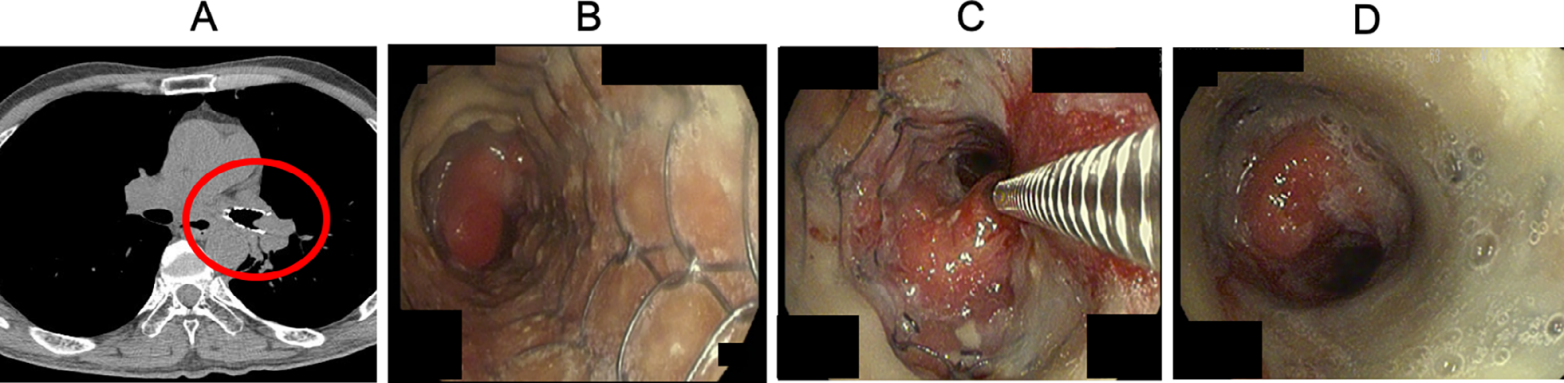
Chest computed tomography showing the narrowing airway at distal portion of the left main bronchus 2 years and 3 months after stent insertion (A). Bronchoscopic examination showing polypoid mass lesion at the distal end of the inserted stent (B). The procedure of bronchial ethanol injection (BEI) therapy with aspiration needle using absolute ethanol (C). Size reduction of polypoid mass lesion after BEI therapy (D)
